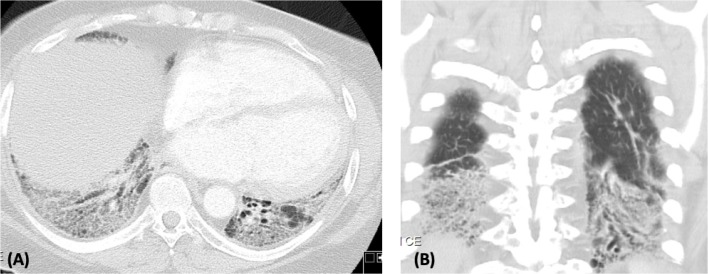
Computer Tomography (CT) axial view (a) and coronal view (b) show honeycombing and lower lobe ground glass opacities suggesting interstitial lung disease.
